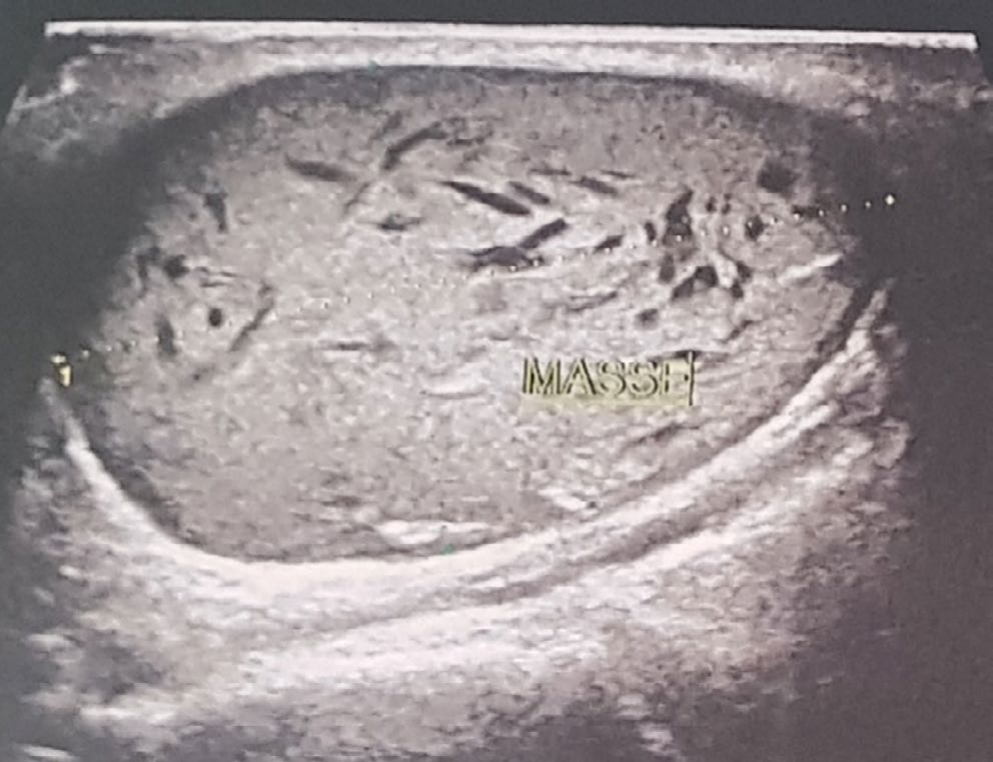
Echographic view: a well‐defined walled hyperechoic heterogenous lesion measuring 34 × 31 × 21 mm with posterior ultrasound reinforcement in the left buccal mucosa region
